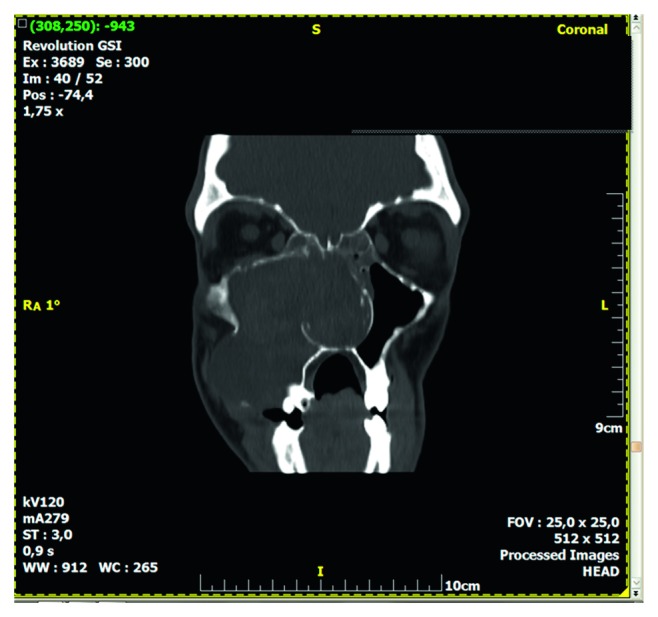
Preoperative CT, coronal plane, bone window.
